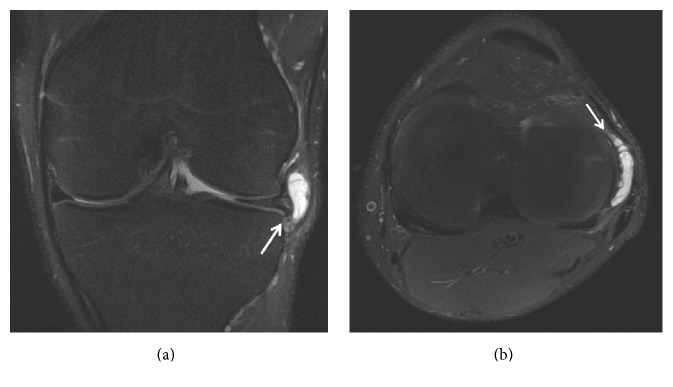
Lateral meniscal cysts: (a) lateral meniscal cysts are usually located at the anterior meniscal horn (coronal T2 FSE MRI sequences); (b) Axial T2 FSE Fat Sat MRI reconstruction showing the lateral meniscal cysts (arrow).
